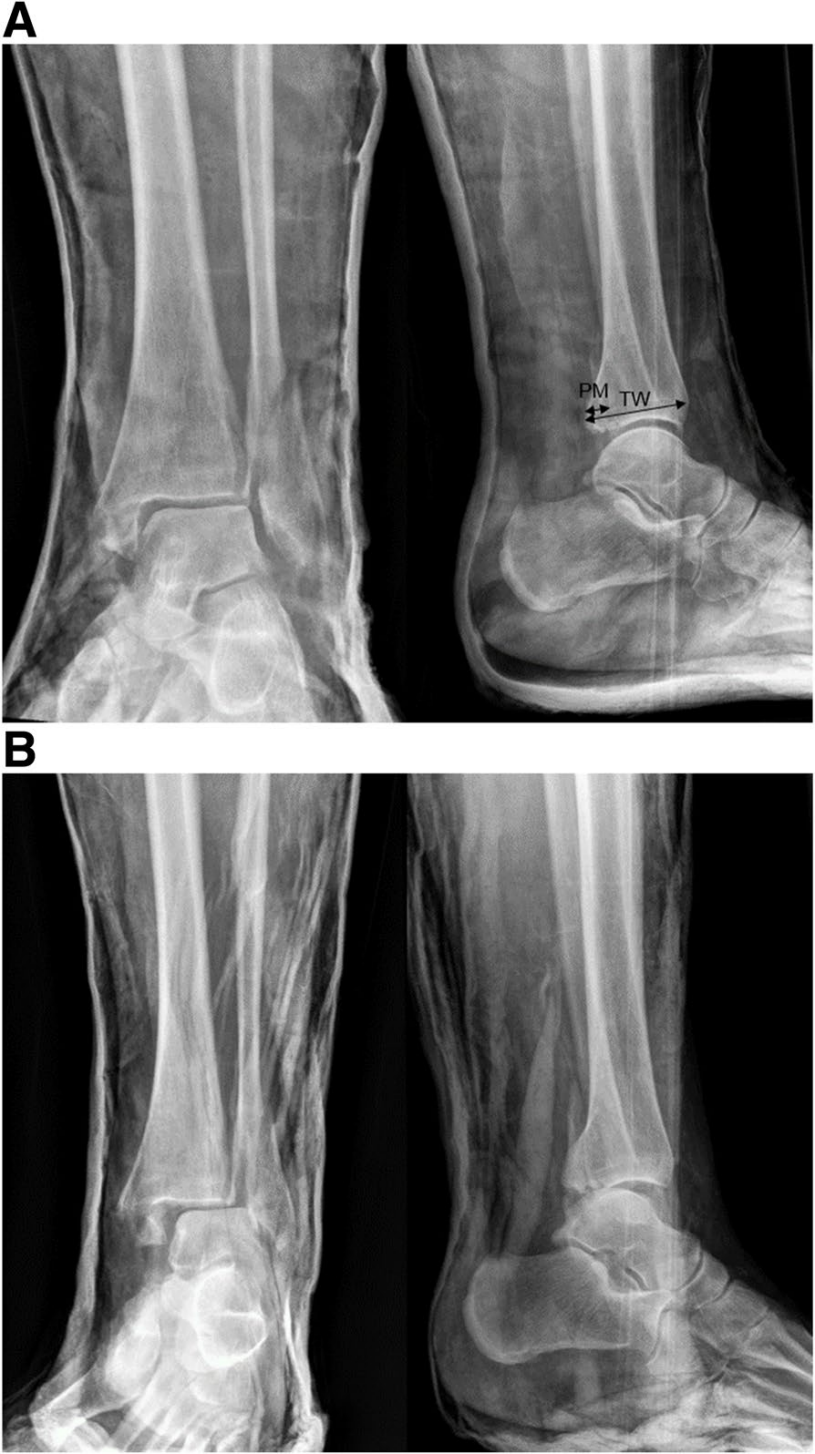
A Anteroposterior and lateral radiograph of a reduced trimalleolar ankle fracture-dislocation temporized in a plaster cast. Measurements were acquired at the level of the epiphyseal scar. The ratio between the width of the posterior malleolar fragment (PM) and the tibial width (TW) defined the posterior malleolar fragment size. In the presented case, the posterior malleolar fragment size was 27%. B Subsequent radiographs demonstrated a loss of reduction
